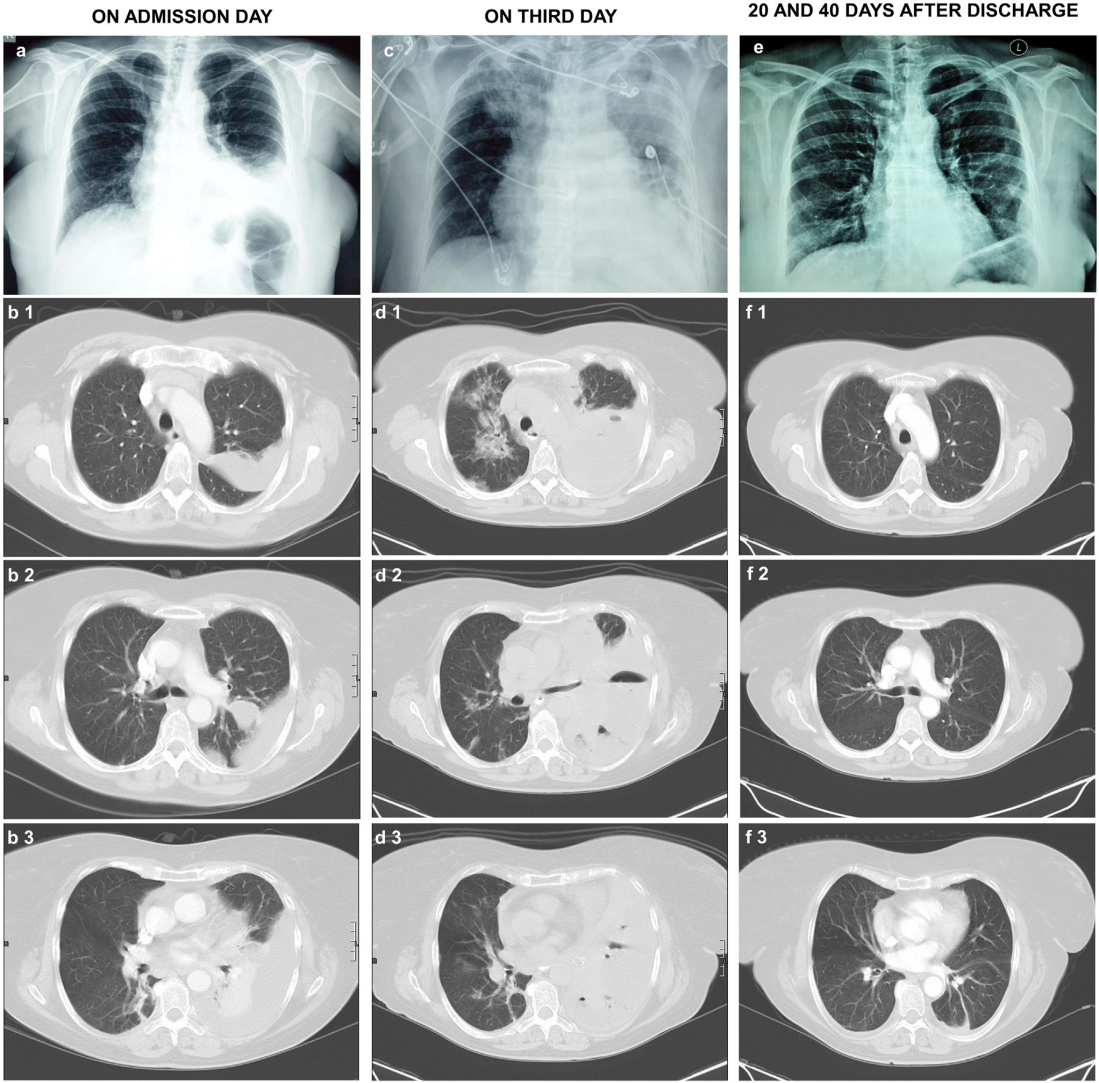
(A) CXR and (B1–B3) CCTs with intravenous contrast material on the admission day exhibit left-sided pleural fluid with consolidation and atelectasis of the left lower lung field. (C) CXR and (D1–D3) CCTs on the third day of admission. They exhibit significant increase of the left-sided pleural effusion with compressive atelectasis of the left lung and development of extensive consolidations in the right lung, especially in the upper lung fields. Small air collections are present in the pleural fluid. The chest drainage tube can be seen on the left of the CXR. (E) CXR 20 days and (F1–F3) CCT 40 days after the patient discharge from the hospital. They show complete resolution of the lung consolidations, limited left pleural thickening, and minimal pleural fluid (1: upper lung fields, 2: middle lung fields, 3: lower lung fields). CXR, chest X-ray; CCT, chest computerized tomography.
